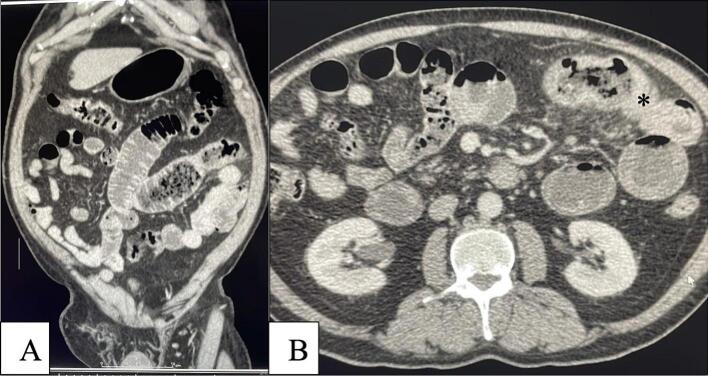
a- Coronal CT scan cut showing small bowel dilatation and the transition point. b- Axial CT scan cut showing small bowel dilatation and the transition point.
